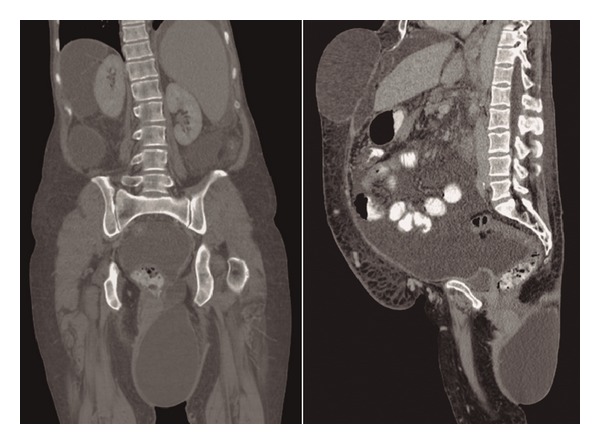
Computed tomography showing a sciatic hernia on the left side with a large hernial sac filled with liquid (ascites).
